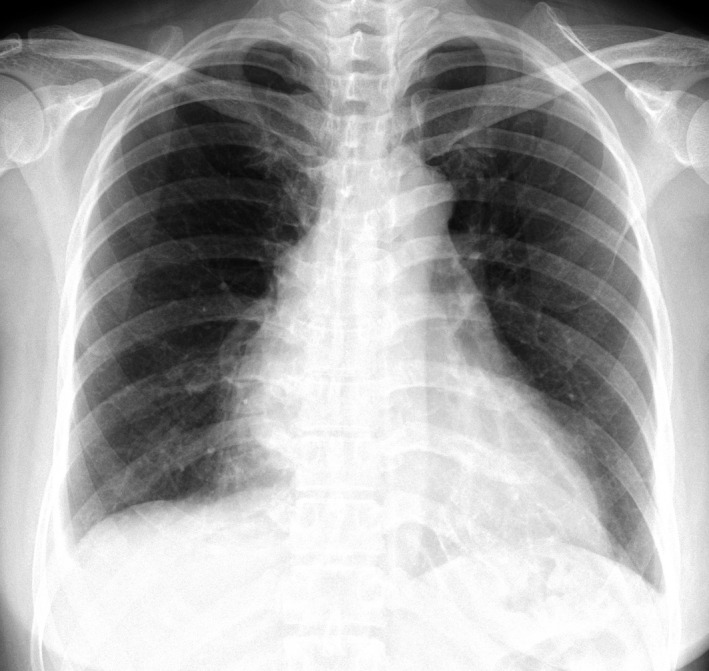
Chest radiograph showed enlarged cardiomediastinal silhouette
